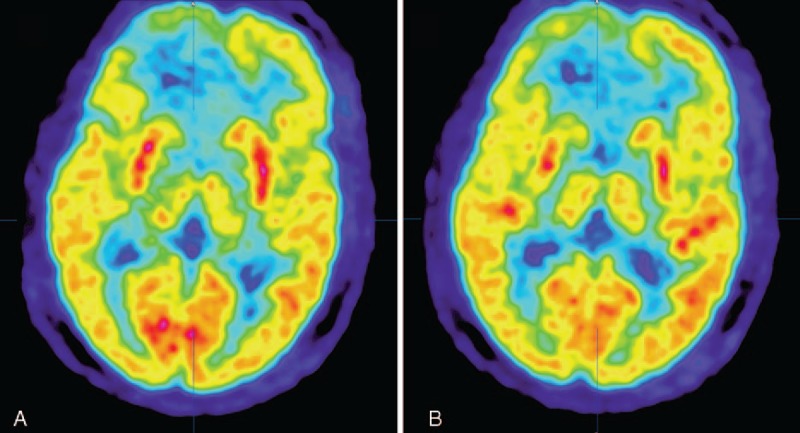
Preoperative brain positron emission tomography–computed tomography (PET-CT) of the patient described in Fig. 3. Because of the formation of necrosis inside the tumor, low 18F-fluorodeoxyglucose uptake in the tumor area was shown. We did not perform another PET-CT examination after the surgery.
